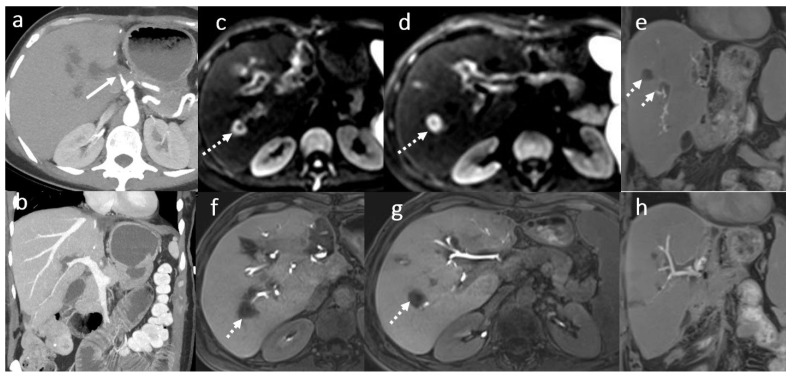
Circumscribed areas of peribiliary liver necrosis due to hepatic arterial thrombosis in a female patient, 49 years old, 3 weeks after OLT: CT angiography (a,b) hepatic arterial thrombosis (arrow). Fluid collection around some of the subsegmental intrahepatic biliary ducts (dotted arrows) in MRI evaluation using diffusion wi (c,d) and 3D T1FS with gadoxetic acid in HBP (e–h).
